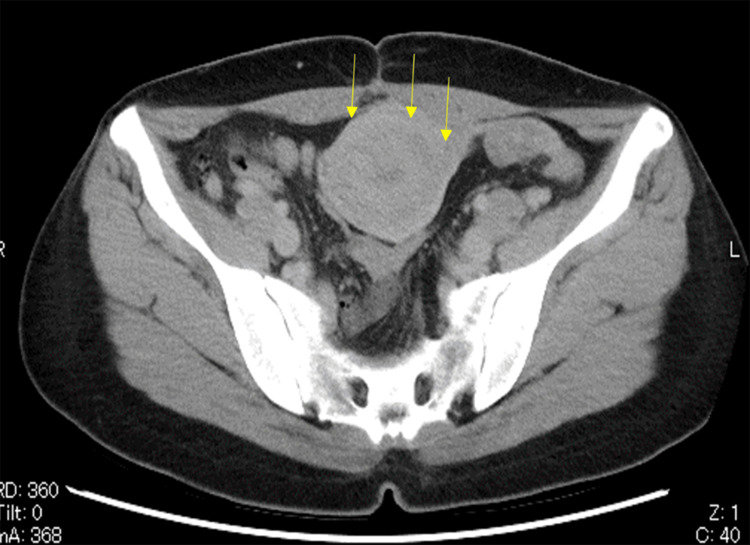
Computed tomography scan findings (Case 7) Findings suggestive of adhesions between the abdominal wall and uterus (arrow).
